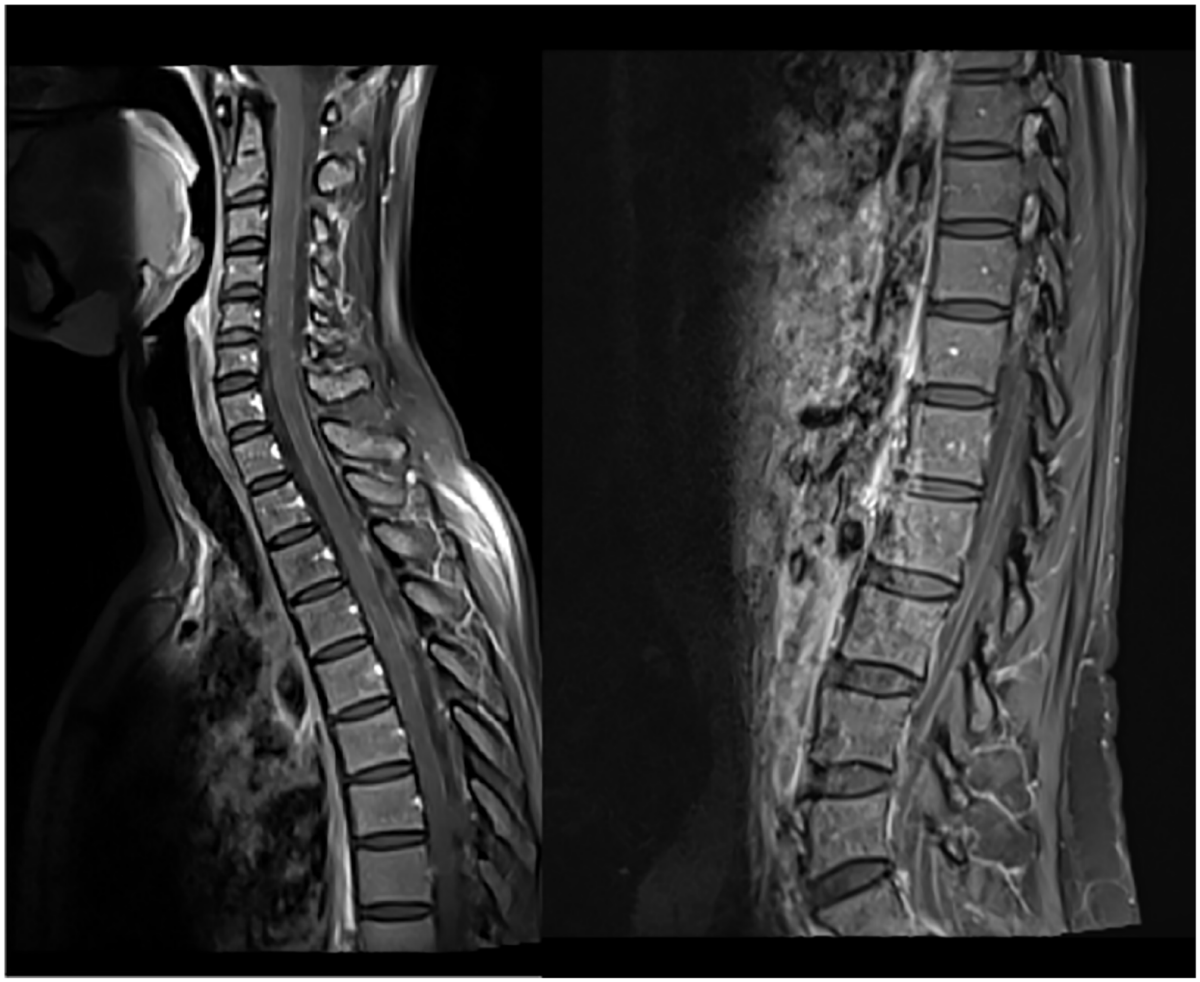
Neuraxis MRI at BM and LMC initial moment of diagnosis. Disseminated LMC (extended to cerebellar fissures, midbrain, brachiocephalic bridge and cranial nerves emergence).
